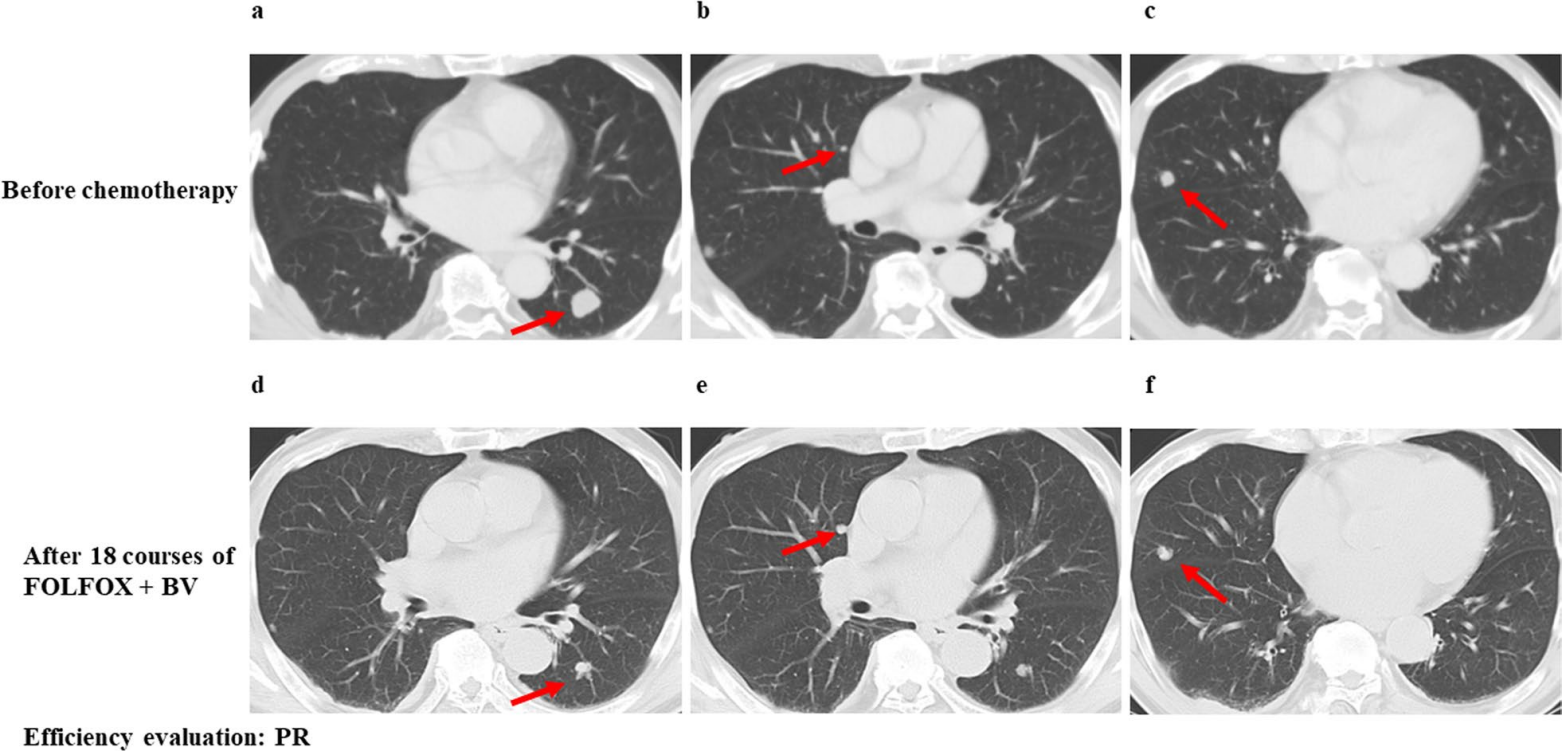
Consecutive CT findings before chemotherapy and after first-line chemotherapy. a–c Multiple lung metastases were revealed. d–f The lung tumors had shrunk after 18 cycles of the FOLFOX + BV chemotherapy regimen had been administered. These arrows indicate lung metastases
